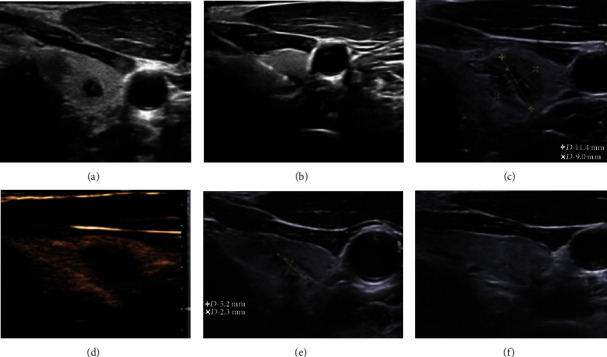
Representative ultrasound images of a PTMC nodule in the left thyroid lobe of a 32-year-old woman before and after ultrasound-guided PLA. (a) Before PLA, the hypoechoic nodule measured 5.1 mm × 3.9 mm × 4.1 mm in size and 42.7 mm^3^ in volume. (b) The laser fiber was inserted into the nodule through the guide needle, and the ablation was started at an output power of 3 W. Immediately, the nodule was completely covered by a patchy hyperecho gasification area. (c) One hour after PLA, the ablation area measured 11.4 mm × 9.0 mm × 10.5 mm, covering an area larger than the original area of the nodule. (d) One hour after PLA, no contrast agent filled the ablated region on CEUS, indicating complete ablation. (e) At 3 months after PLA, the ablation area was decreased to 5.2 mm × 2.3 mm × 3.1 mm. (f) At 6 months after PLA, the ablation area had been completely absorbed. No recurrence or lymph node metastasis was found during 5 years of follow-up.
